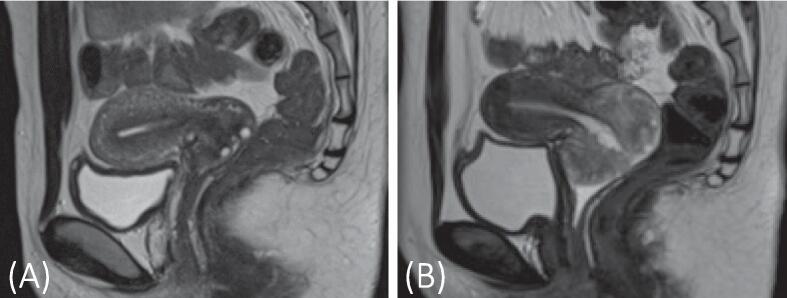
(A) T2-weighted MRI image from the initial gynecologic visit of the patient, indicating no suspicious findings in the cervix. (B) T2-weighted MRI image captured 17 months later, demonstrating a high signal intensity in the cervix, suggestive of LEGH. MRI, magnetic resonance imaging; LEGH, lobular endocervical glandular hyperplasia.
